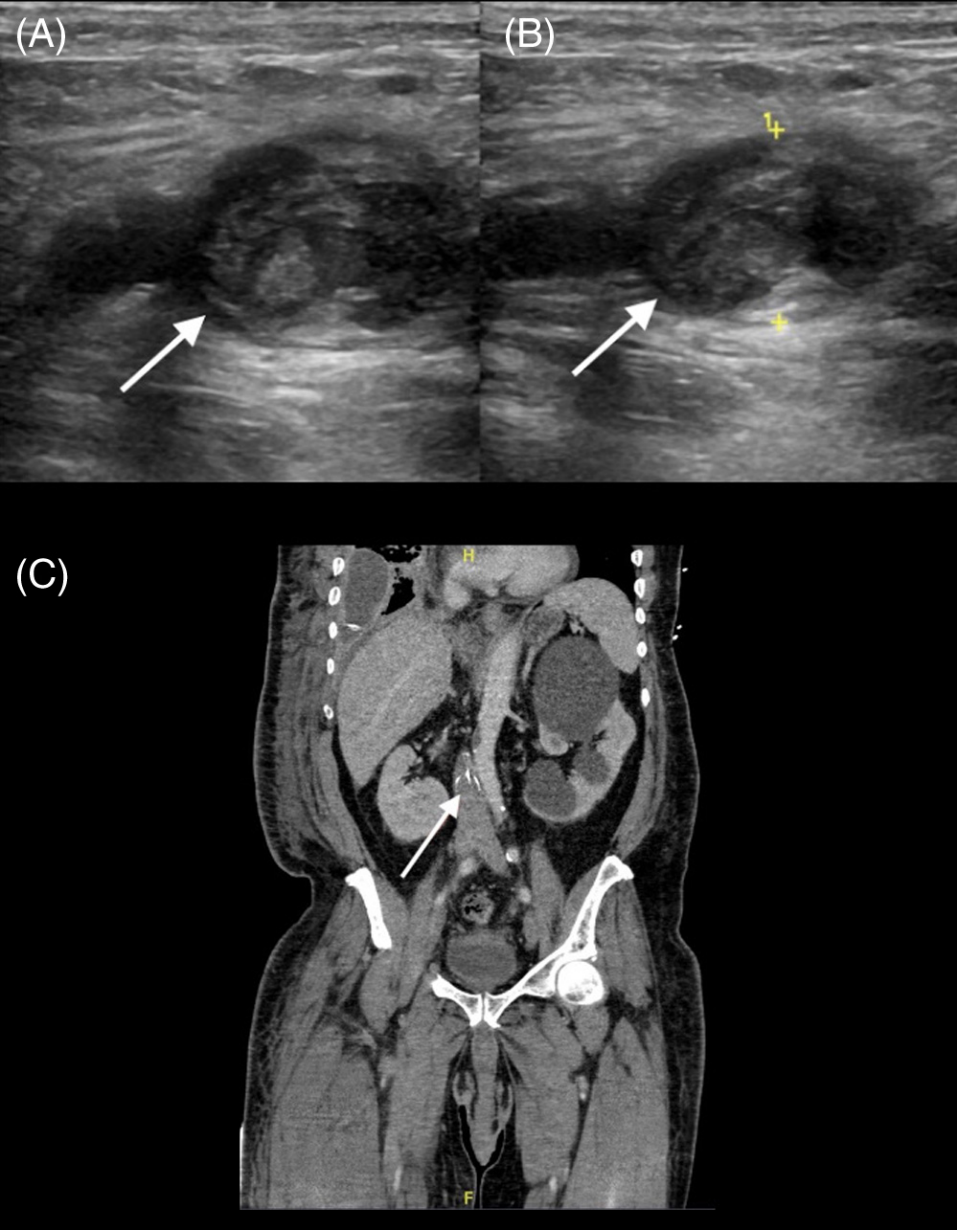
Top: Ultrasound of the right common femoral vein demonstrating clot (A) with incompressibility of the vein (B). Bottom: Computed tomography of the abdomen and pelvis demonstrating thrombosis of the inferior vena cava to the level of implanted inferior vena cava filter (C).
